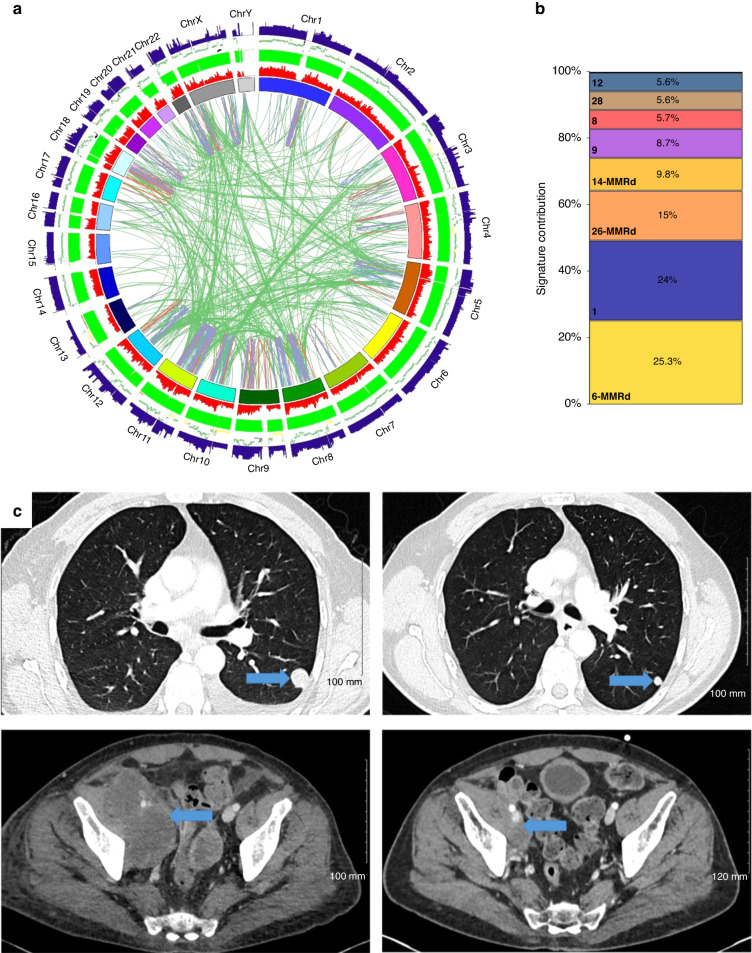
Therapeutic impact of WGS in sarcoma (case 31). a Circos plot of WGS showing strong mutational burden of indel variants (green track). b Signature breakdown showing strong mismatch repair signature. c CT scan images of a 56-year-old male with progressive lung and pelvic metastases from UPS following two previous lines of chemotherapy showing excellent partial response to the immune checkpoint inhibitor pembrolizumab.
